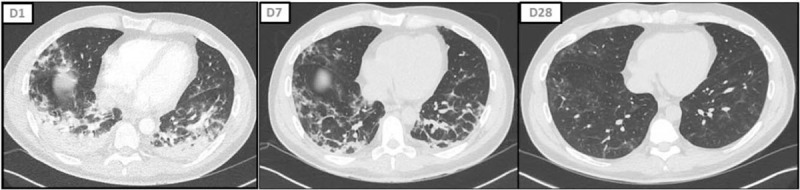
Chest computed tomography scan evolution of a 42-old patient treated with tocilizumab (intravenous, 600 mg).
